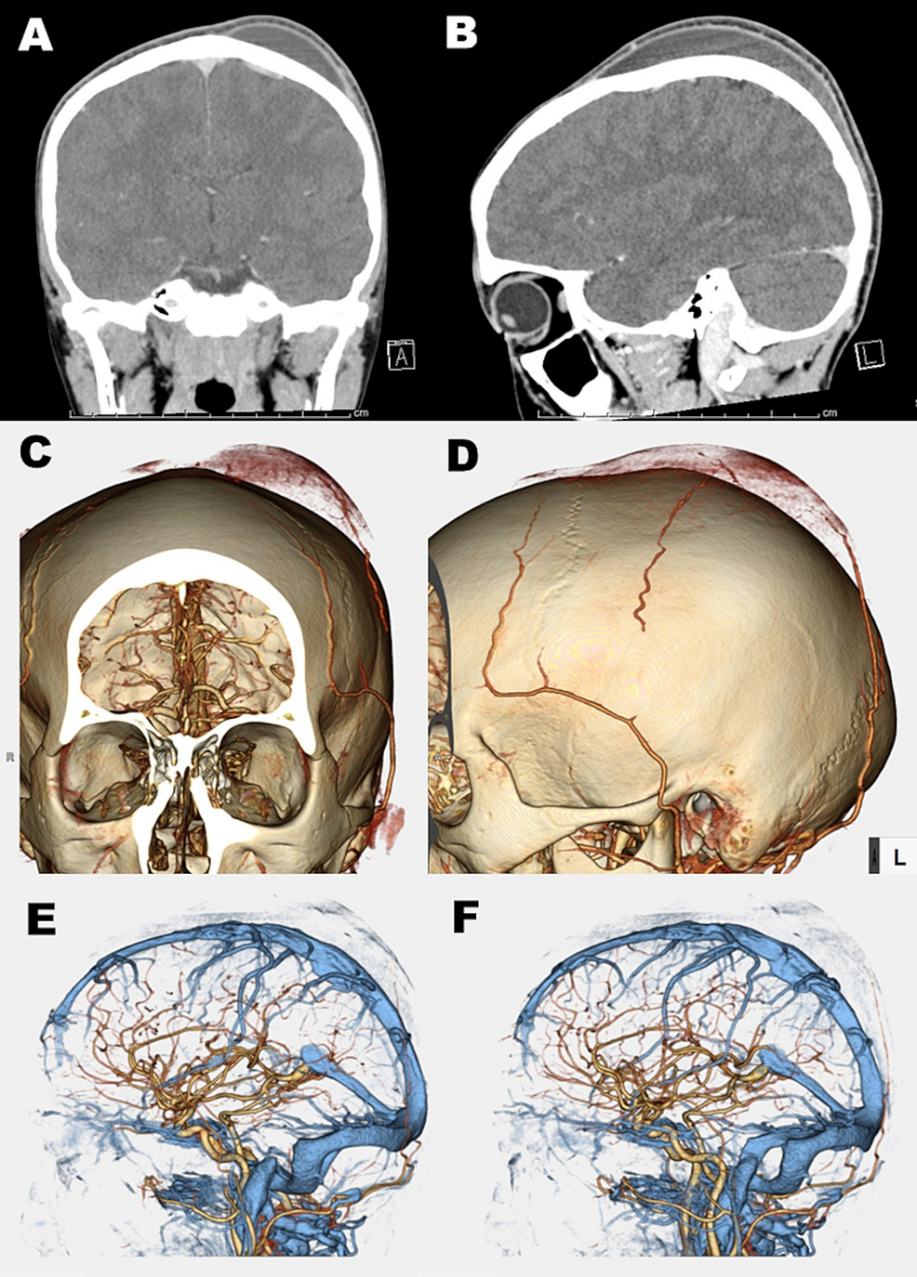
Postcontrast CT scan images and three-dimensional CT angiography. Postcontrast CT scan images show enhancement along the subperiosteal hematoma cavity (A, B), which is confirmed by three-dimensional CT angiography (C, D). Damage of the superior sagittal sinus or adjacent veins was not demonstrated (E, F).
